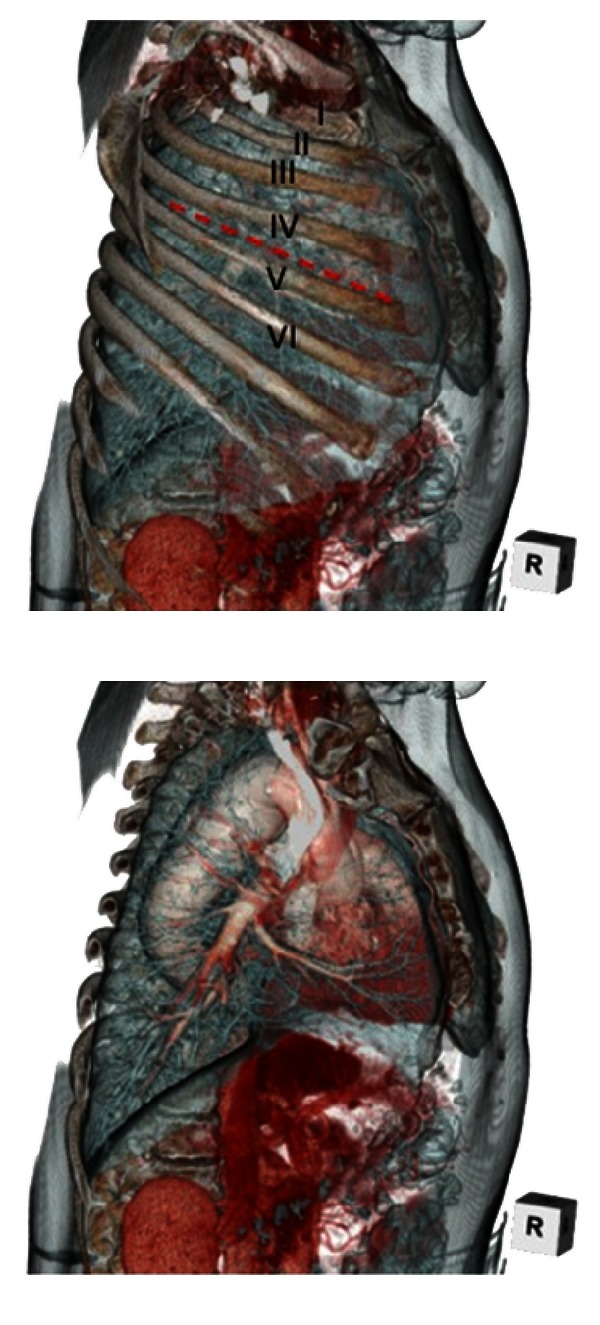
3D-CT imaging, scrolling from superficial to deep layer view with chest wall including the ribs, showed the surgical view and the optimal approach through a posterolateral thoracotomy with the fourth intercostal incision.
